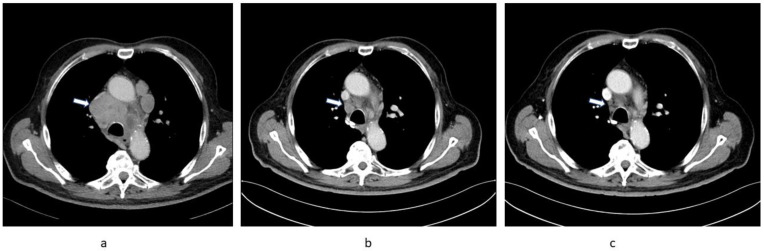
Sequential chest CT images of Patient 1 showing regression of substantial mediastinal lymphadenopathy (arrow). (a) 28 August 2020, (b) 15 February 2021, (c) 18 May 2021.
